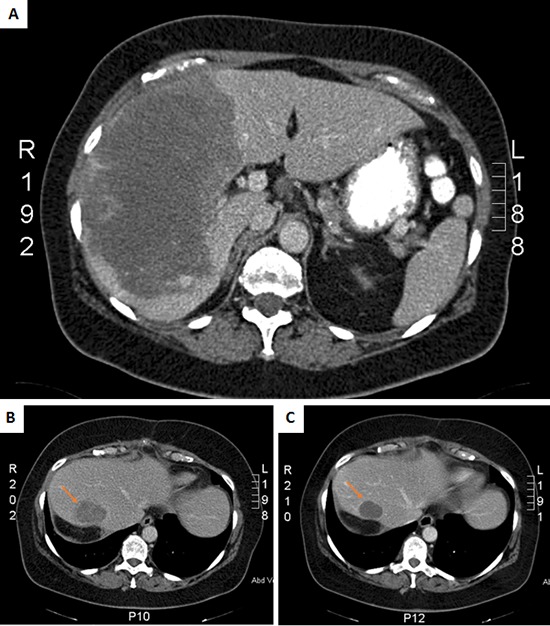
CT scans of primary tumor at diagnosis A. and the recurrent liver tumor at baseline B. and after 2 months treatment with sunitinib C.
